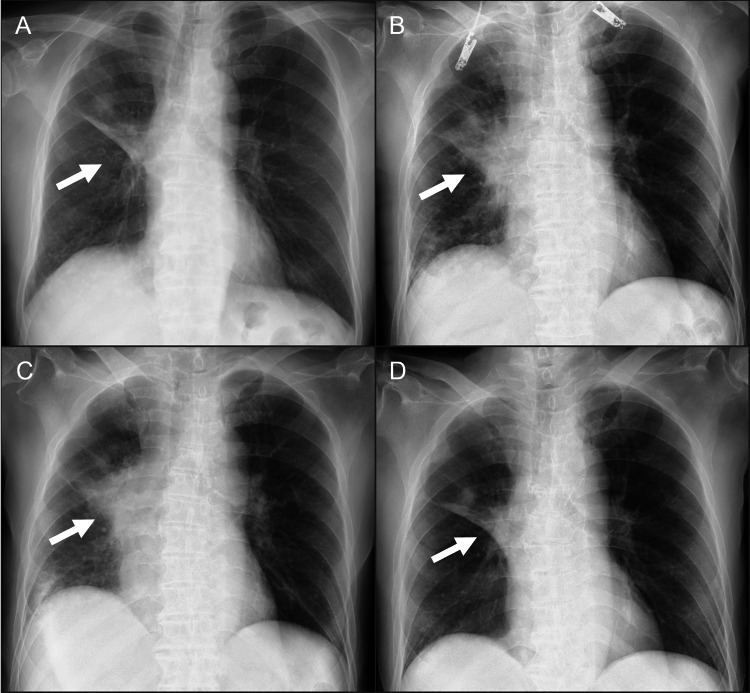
Radiographic course on chest X-ray. The right hilar mass increased in size on days two (B) and four (C). On day seven (D), the mass had decreased in size. Arrows indicate the areas of interest. (A) Chest radiograph on day zero. (B) Chest radiograph on day two. (C) Chest radiograph on day four. (D) Chest radiograph on day seven.
